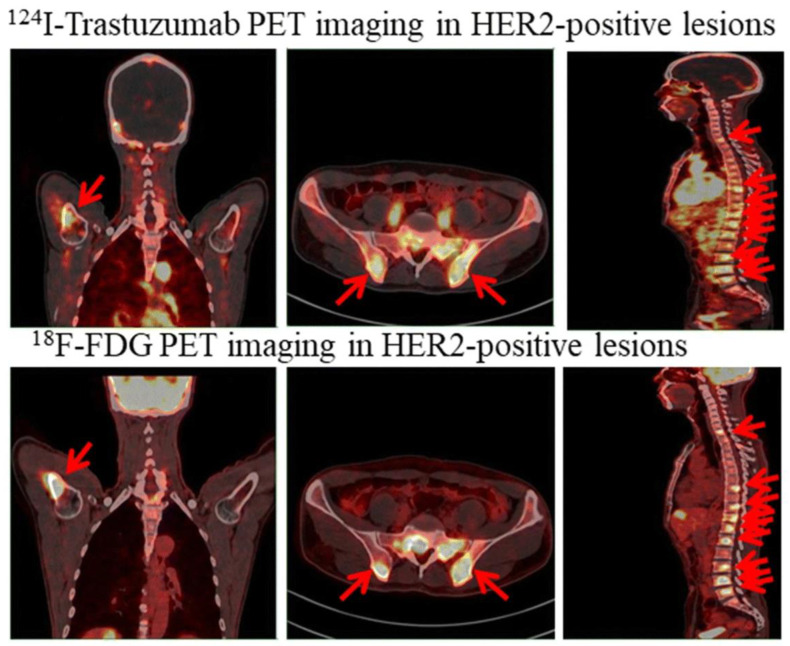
Detection of HER2-positive lesions with ^124^I-trastuzumab and standard ^18^F-FDG PET imaging in humeral, bilateral ilium, and spinal metastases. Arrows point to HER2-positive gastric cancer lesions. Reprinted/adapted with permission from Ref. [136].
